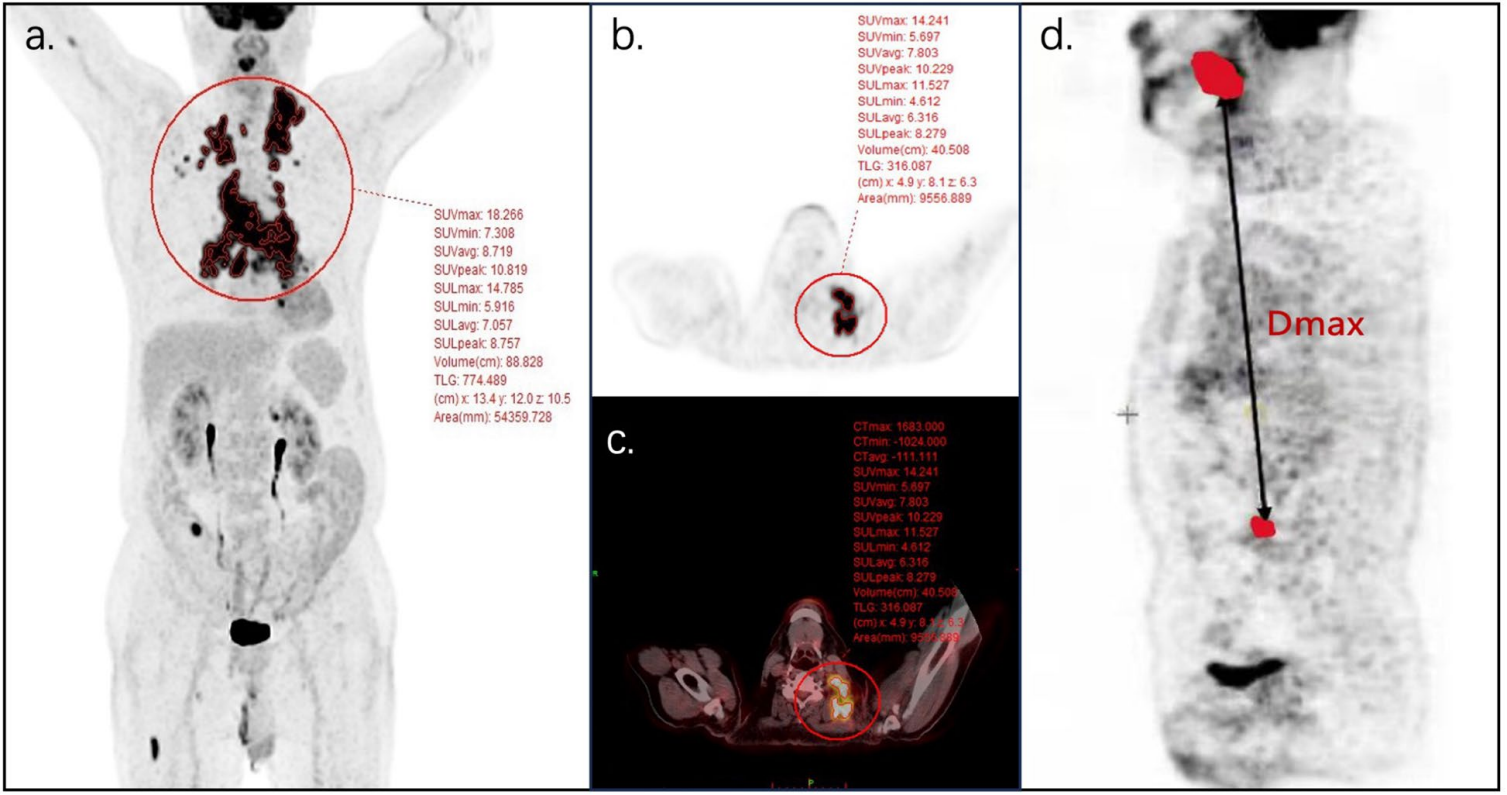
Three semiquantitative parameters in coronal (a) and axial coronal images(b, c) of FDG PET/CT from one patient: SUV, MTV and TLG. Dmax, the distance between two lesions that are furthest apart in sagittal images (d) of FDG PET/CT
